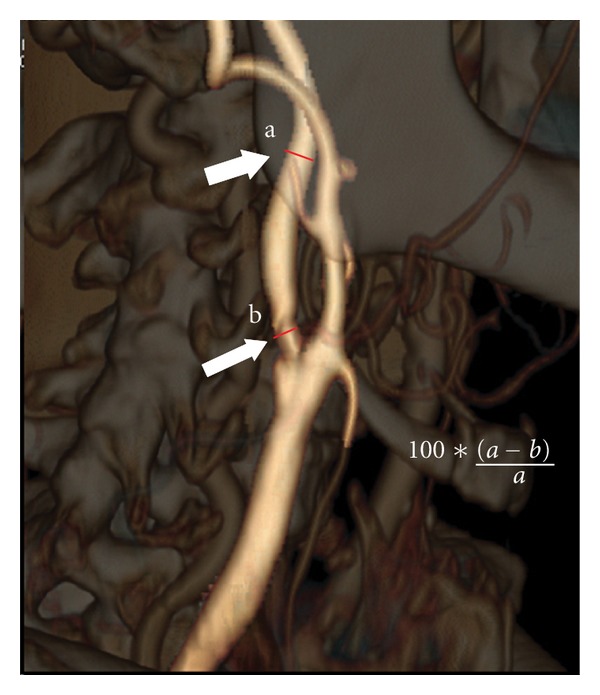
Volume rendering CT angiography showing measurement of internal carotid artery stenosis. Following the NASCET criteria, a measure of the ratio at the point of greatest stenosis (small white arrow) and at the normal part of the artery beyond the carotid bulb (big white arrow) was performed.
